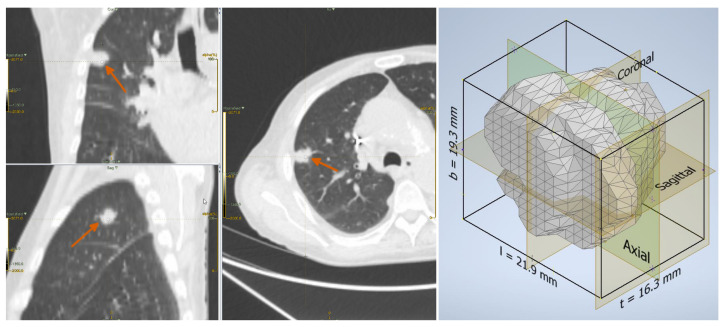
Adenocarcinoma in a 76-year-old man: lesion on the CT scan (left) and the reconstructed three-dimensional volume within the axis-aligned bounding box (right).
